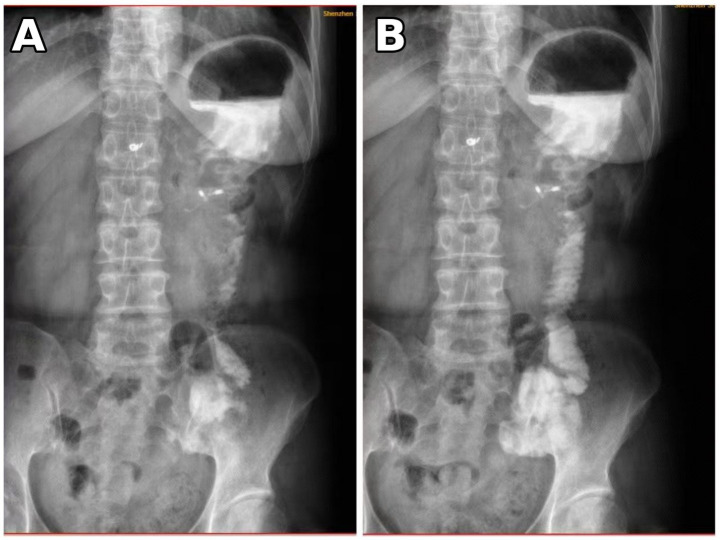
One-month post-treatment contrast study. (A) Radiograph obtained 30 min after oral contrast administration, showing improved passage of contrast into the efferent limb. (B) Radiograph obtained 3.5 h after oral contrast administration, showing smooth downstream transit without delayed gastric emptying.
